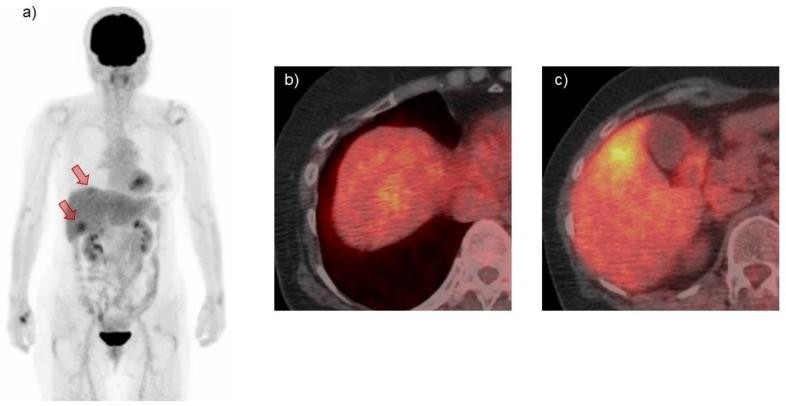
FDG-PET and computed tomography detected increased uptake in the liver tumor lesions (arrows), with no uptake in other organs (a–c).
